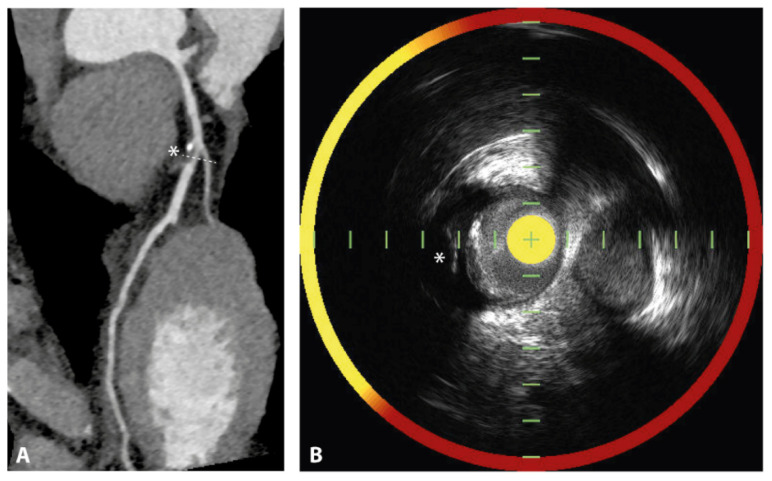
Vulnerable plaque on CTCA. (A) displays the left anterior descending artery on computed tomography coronary angiography (CTCA) of a patient with stable angina pectoris. A low-attenuation plaque is demonstrated with spotty calcification. (B) displays the intravascular ultrasound (IVUS) images with near-infrared spectroscopy (NIRS) of the same patient, corresponding with the cutline in (A) around the bifurcation. The IVUS image displays an echolucent plaque (*) with deep echo attenuation and small calcium deposits. The NIRS chemogram colors yellow, indicating the presence of a large lipid core. Image obtained from Van Veelen et al. Reviews in Cardiovascular Medicine 2022. CC-BY [4.0] [9].
